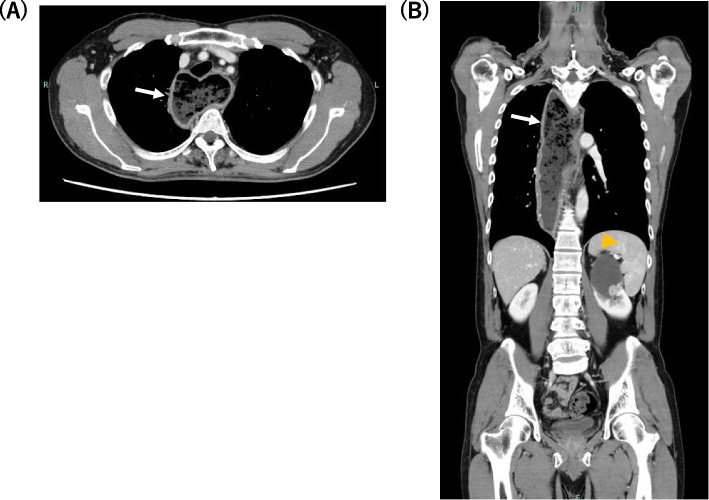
A Axial computed tomography (CT) image showing the enlarged esophagus filled with residue (arrow). B Coronal CT image showing dilation of the upper part and narrowing of the lower part of the esophagus, presenting a bird beak shape (white arrow). The left renal tumor is also seen (yellow arrowhead)
